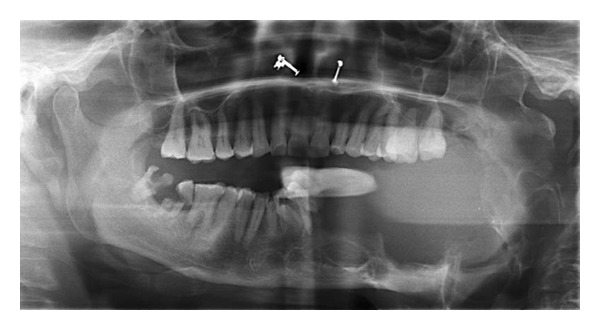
Radiographic examination revealed a multilocular radiolucency, extending from left condyle and coronoid process to right central and lateral incisors with thinning of lower border of mandible.
